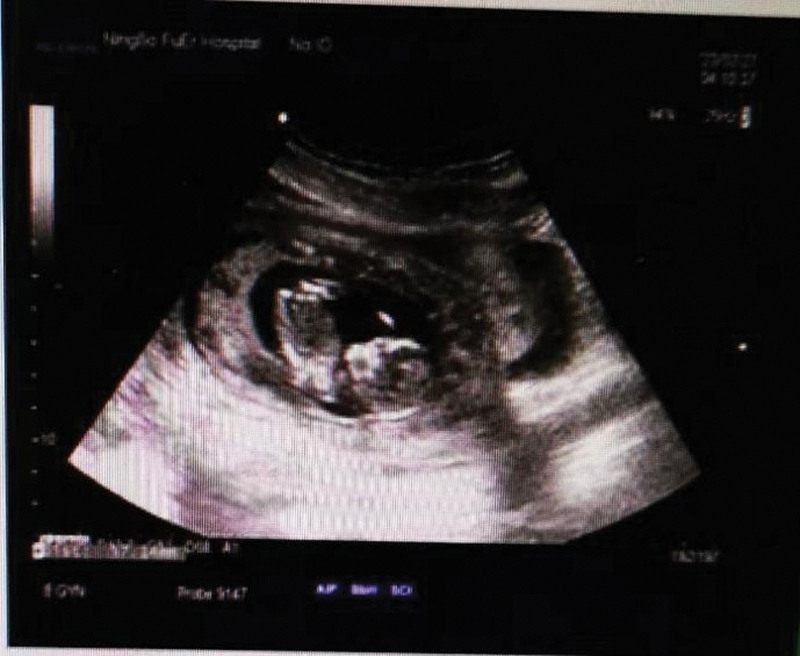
Ultrasound, fetus in the uterus. Liquid was stored in anterior recess and right iliac fossa.
